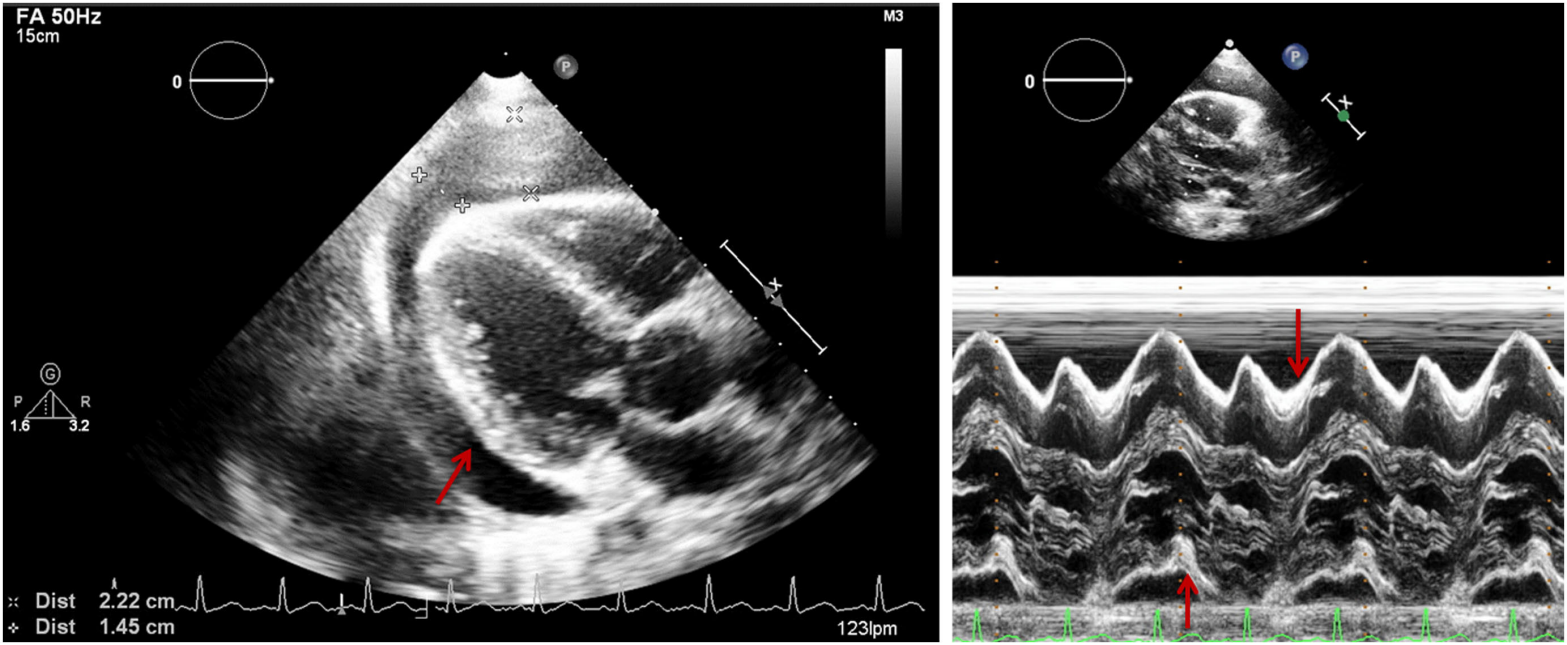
Parasternal long axis (two-dimensional on the left and M-mode on the right) in a patient with pericardial effusion (PEff). Arrow shows echo-free signal from pericardial fluid. M-mode shows PEff only during systole.
